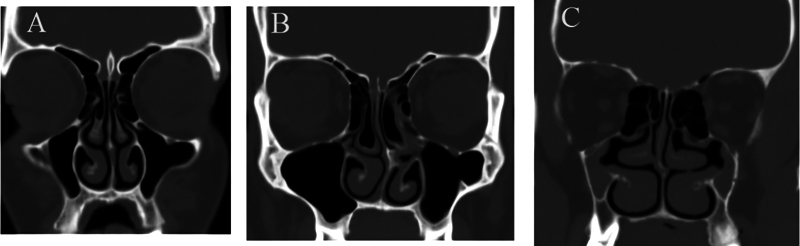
Computed tomography scans showing the types of uncinate process (UP); (
